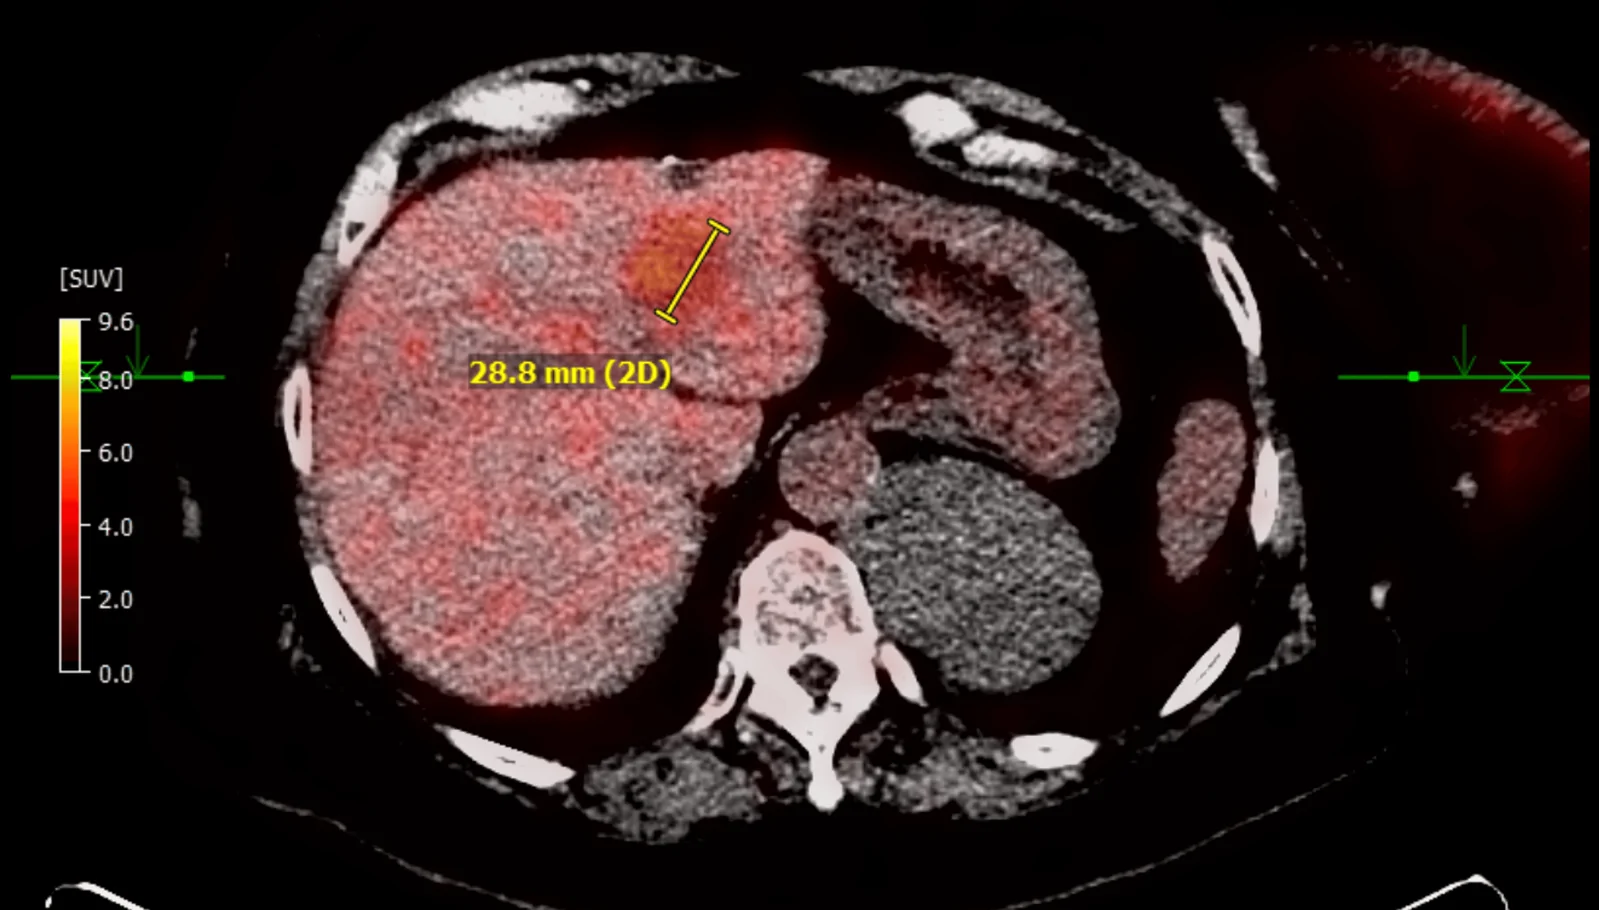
PET scan showed an FDG-avid lesion within the liver measured at 28.8 mm
